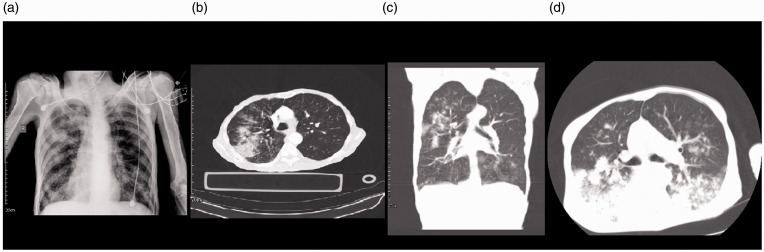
Chest imaging of a 40-year-old male with COVID-19. 2 A: Chest X-Ray
